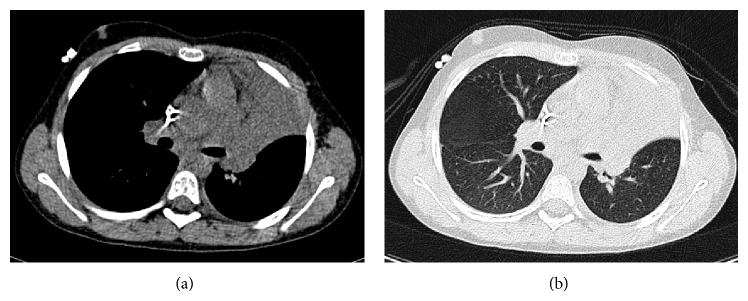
CT scan images of the chest performed on day 9 after transplant. (a) demonstrates complete collapse of the left upper lobe with abrupt cut-off of the left upper lobe bronchus and (b) absence of air bronchograms within the collapsed lung.
